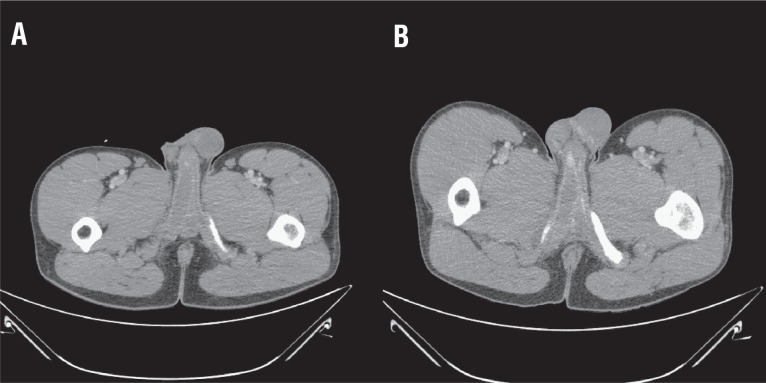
Computed tomography image of patient with partial priapism of the proximal right corpus cavernosum. A) Initial imaging at first presentation to the emergency room; B) Imaging three days later showing well-defined transverse membrane separating the proximal thrombosed and distal non-thrombosed sections of right corpus cavernosum.
